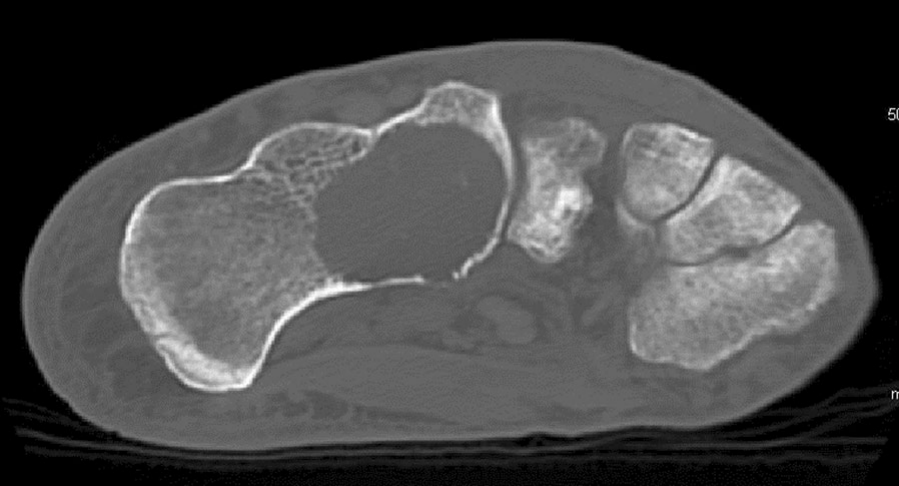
Axial CT scan of lesion preoperatively displaying concern for cortical destruction
